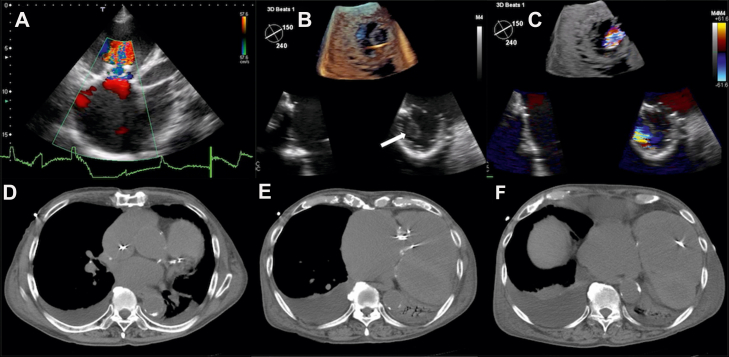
Multimodal imaging demonstrating tricuspid stenosis and right atrial enlargement. Transthoracic echocardiography (A) demonstrates accelerated flow across the tricuspid valve into the right ventricle. Transesophageal echocardiography (B, C) shows incomplete opening of the bioprosthetic valve, with only 1 leaflet visualized as open (*white arrow* in panel B). Chest computed tomography images after temporary pacemaker insertion (D–F) reveal a markedly enlarged right atrium.
